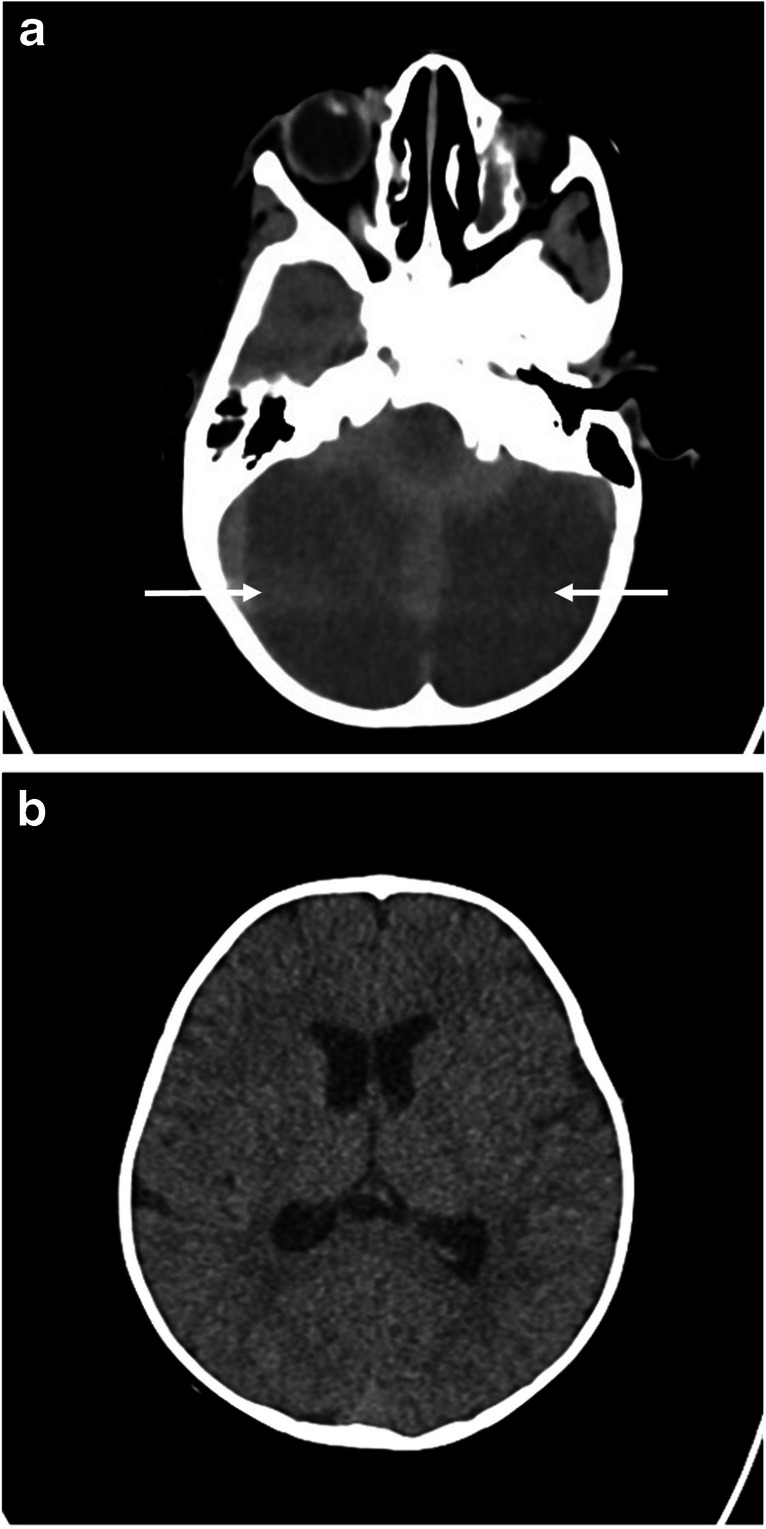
Two-year-old girl found unresponsive after fentanyl ingestion. a Axial noncontrast CT head at the level of the posterior fossa shows bilateral diffuse cerebellar hypodensity suggestive of diffuse edema or infarction. b Mild ventriculomegaly is noted at the level of the lateral ventricles
